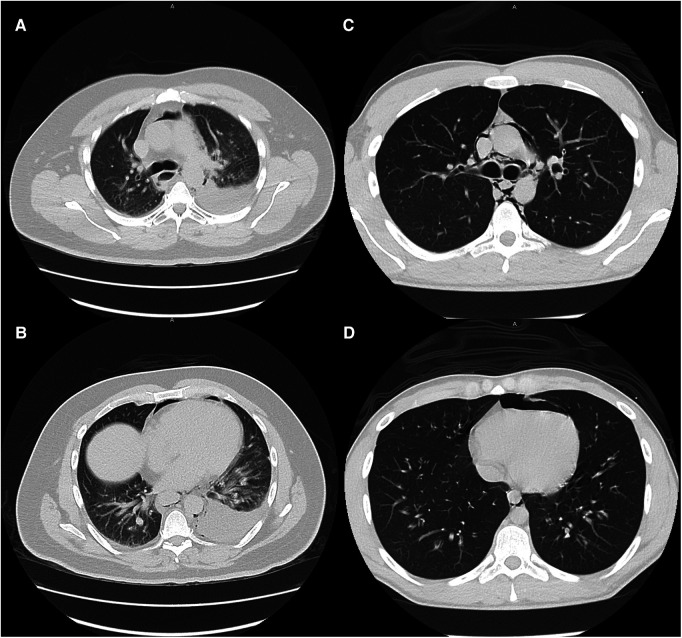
Typical CT scan findings of esophageal perforation (patient 13, images A and B) and marijuana-associated pneumomediastinum (patient 27, images C and D) at similar levels. Moderate to large pleural effusions are typically associated with esophageal perforation. None of the marijuana-associated sPMD patients presented with pleural effusions.
